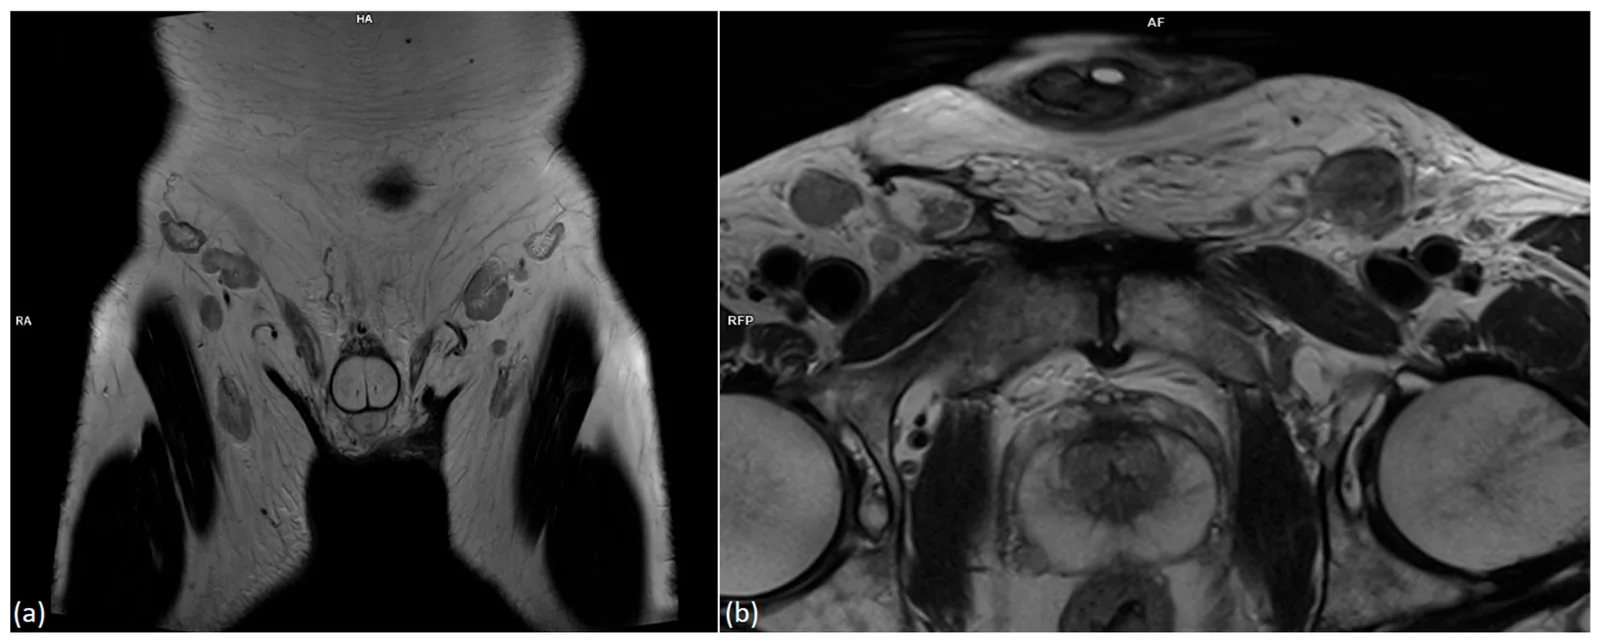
Inguinal lymph node assessment using MRI: (a) morphologically suspicious inguinal nodes that were pathologically negative, representing a false-positive MRI finding; (b) pathologically metastatic inguinal nodes, which may be non-palpable when small.
